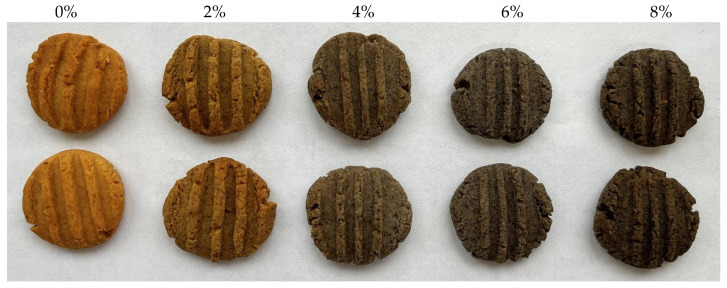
The appearance of cookies control, 2%, 4%, 6%, and 8% of CT flower.
